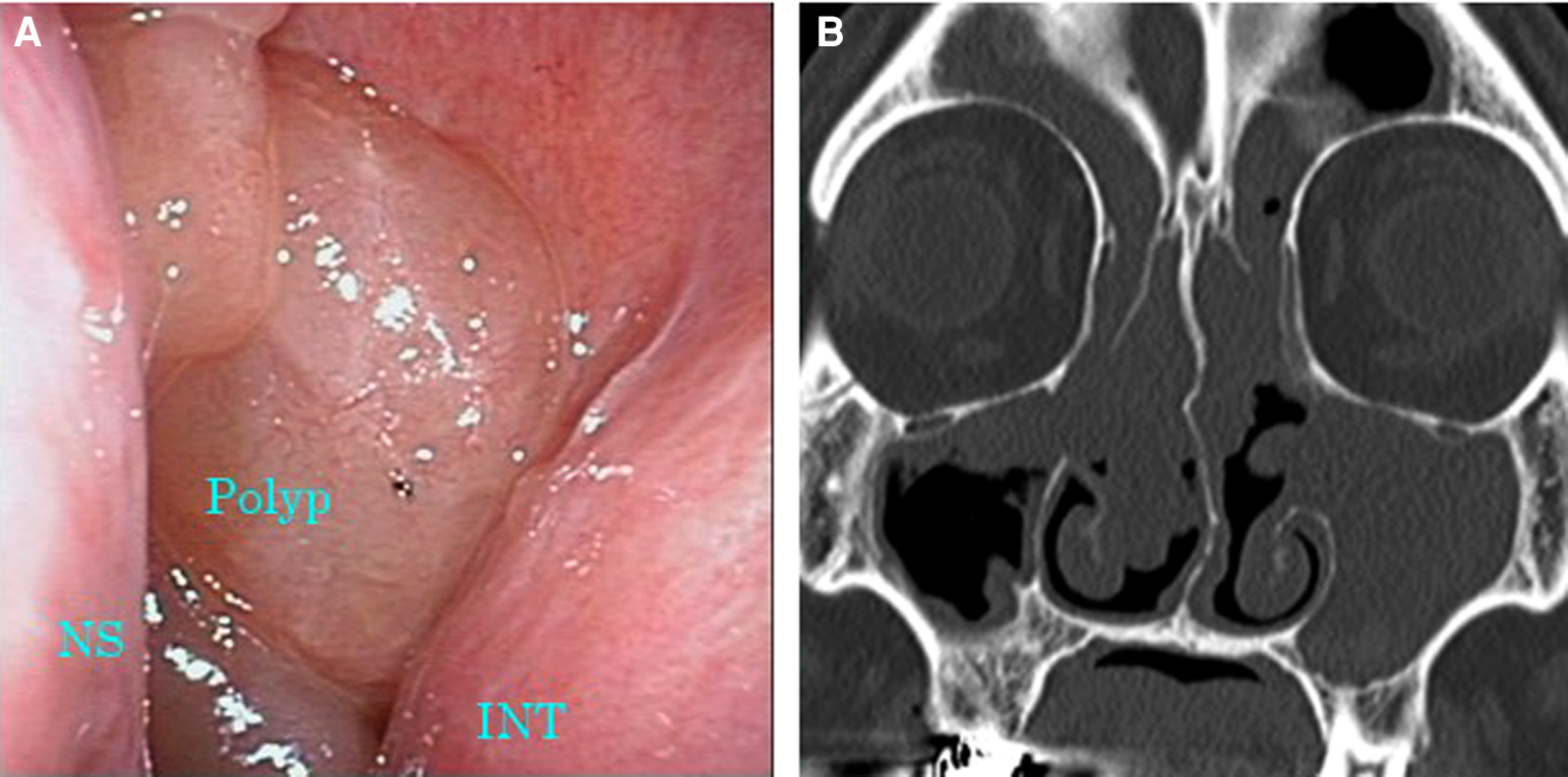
Images of nasal endoscopy and sinus CT 6 months after the initiation of dupilumab therapy. (A) Nasal polyps occupying the common nasal meatus were still observed. (B) Soft tissue density filled all sinuses on both sides. CT, computed tomography; INT, inferior nasal turbinate; NS, nasal septum.
